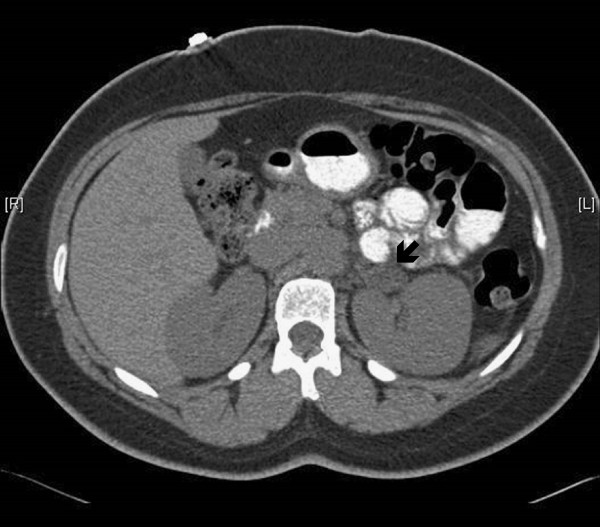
Adrenal CT scan of patient show left adrenal adenoma (see arrow).
